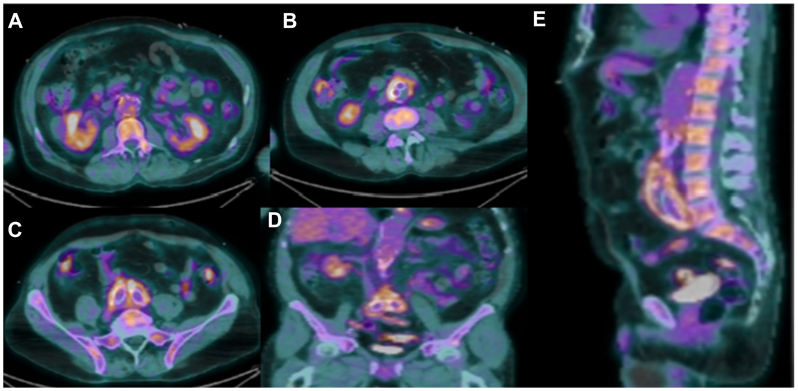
Positron emission tomography with computed tomography (PET-CT) preoperative scan showing fluorodeoxyglucose avidity along the entirety of the graft with adjacent inflammatory stranding. (A) Axial image, at the level of the renal arteries. (B and C) Axial images showing uptake of limbs of the aortic prosthesis. (D and E) Coronal and sagittal views.
